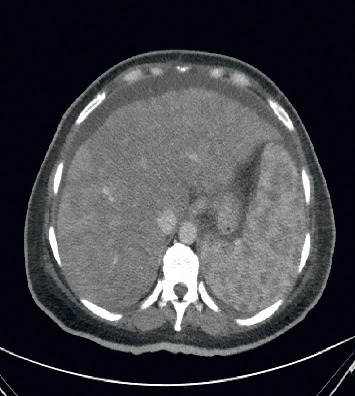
Computed tomography of the abdomen with contrast showing hepatosplenomegaly, numerous hypodensities in the spleen and ascites.
